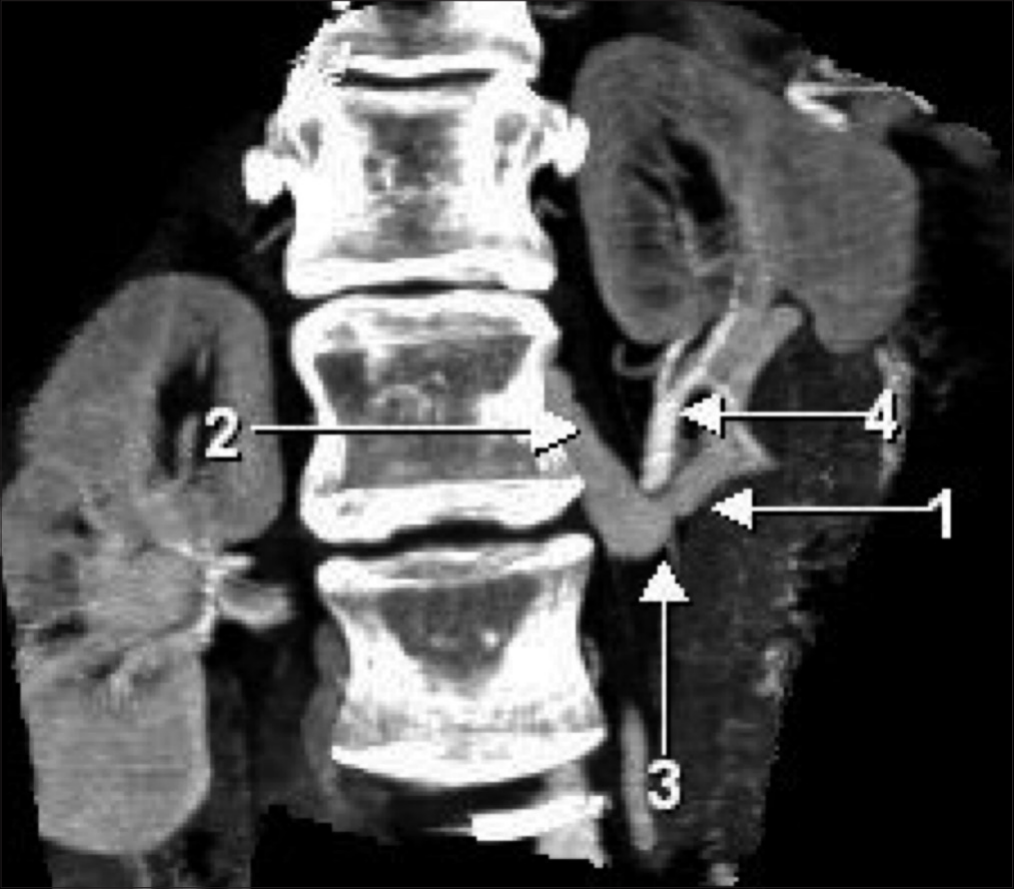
Coronal reformatted maximum intensity projection CT scan shows the varicosity of the communicating vein between the left renal vein and the left ascending lumbar vein in close proximity to the left renal artery. (The arrows point to the same structures as in Figure 3)
